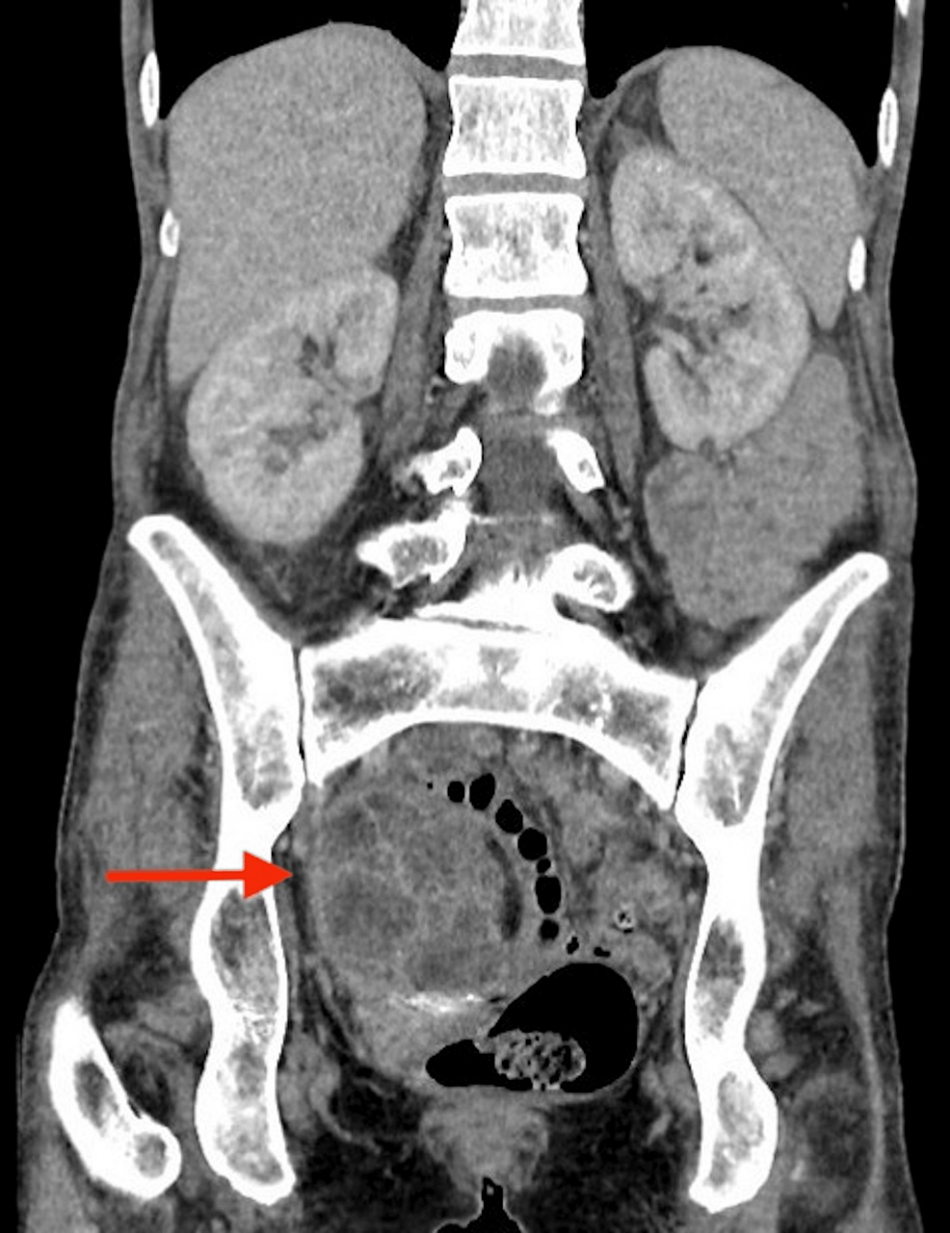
Coronal section image of CT abdomen demonstrating right adnexal mass Mixed density right adnexal mass inseparable from right ovarian dermoid and the uterus, abutting the bowel.
